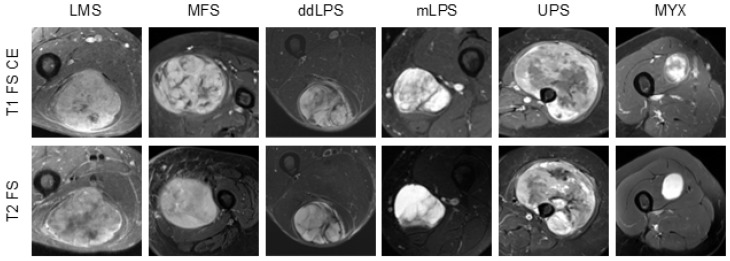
Representative T1-weighted fat-saturated contrast-enhanced and T2-weighted fat-saturated MRI images of six different STS subtypes: Leiomyosarcoma (LMS), Myxofibrosarcoma (MFS), Dedifferentiated Liposarcoma (ddLPS), Myxoid Liposarcoma (mLPS), Undifferentiated Pleomorphic Sarcoma (UPS), and Myxoma (MYX).
